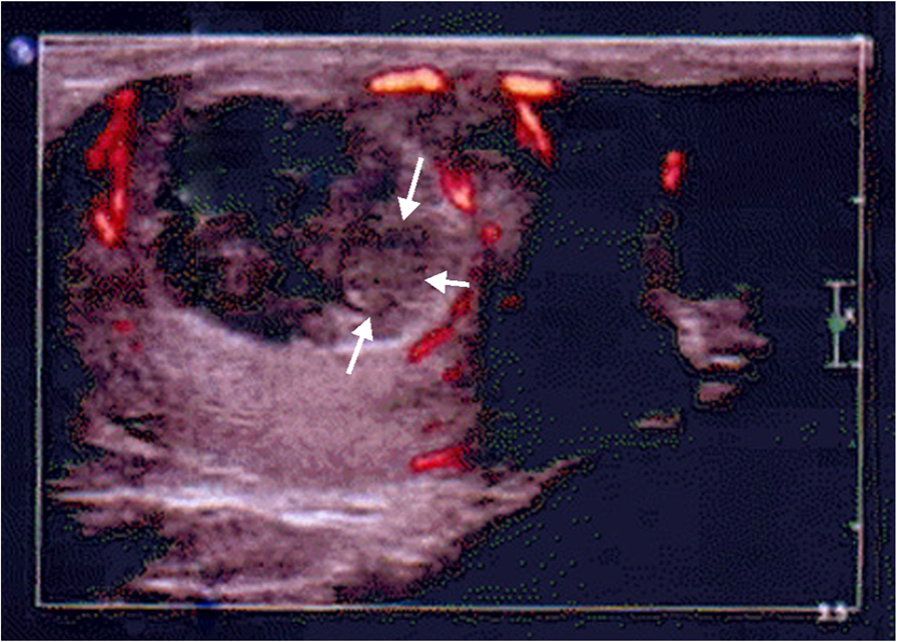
Testicular ultrasound showing an 18 mm × 12 mm × 25 mm heterogeneous hypoechogenic mass localized to the upper pole of the right testis (*arrows*)
